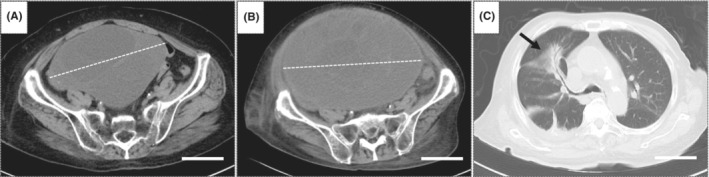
Diagnostic imaging on admission. (A) A non‐contrast computed tomography (CT) scan of the abdomen 15 months prior to admission shows a cystic tumor of the ovary, 19 cm in diameter. The white dotted line indicates the diameter of the tumor. (B) A non‐contrast CT scan of the abdomen at admission shows a cystic tumor of the ovary, 24 cm in diameter. The white dotted line indicates the diameter of the tumor. (C) The arrow indicates a high‐density area along the bronchus in the upper lobe of the right lung in the non‐contrast CT scan of the chest at admission. Scale bars = 5 cm (A–C).
